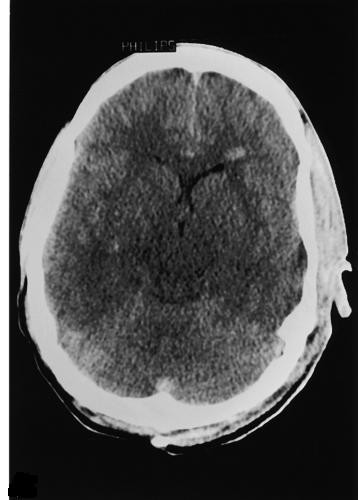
A computed tomography scan at admission showing mild brain edema.
